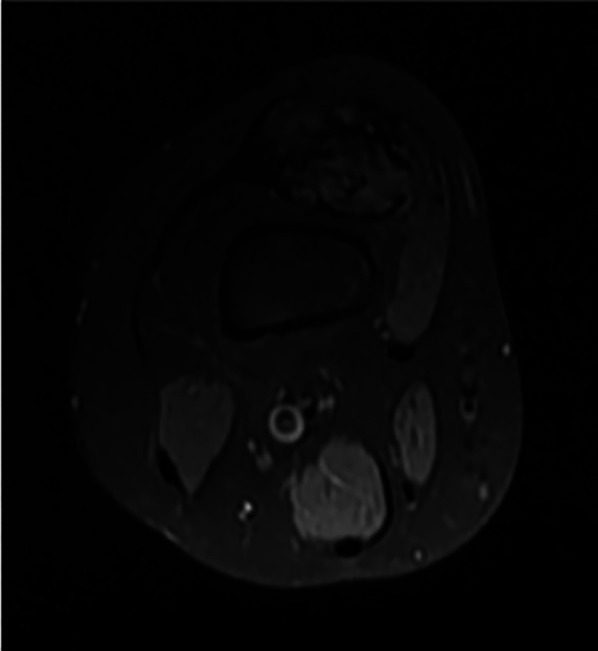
Ax T1 FS knee MRI - typical hamartoma.
